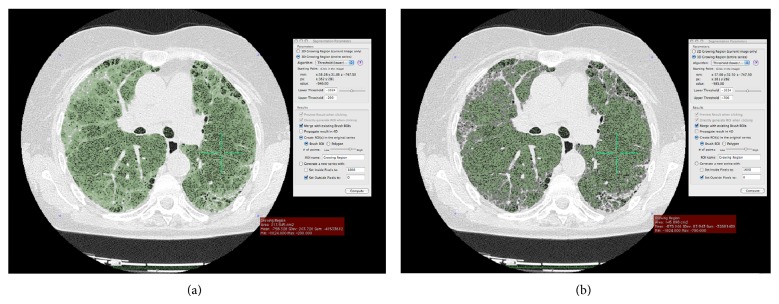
Representative sequences of the OsiriX segmentation process. We have developed the pulmonary fibrosis fraction by the following formula: total HRT lung volume (−1.024 to −200) – nonfibrotic HRCT lung volume (−1.024 to –700) divided by total HRT lung volume (−1.024 to −200) multiplied by 100.
